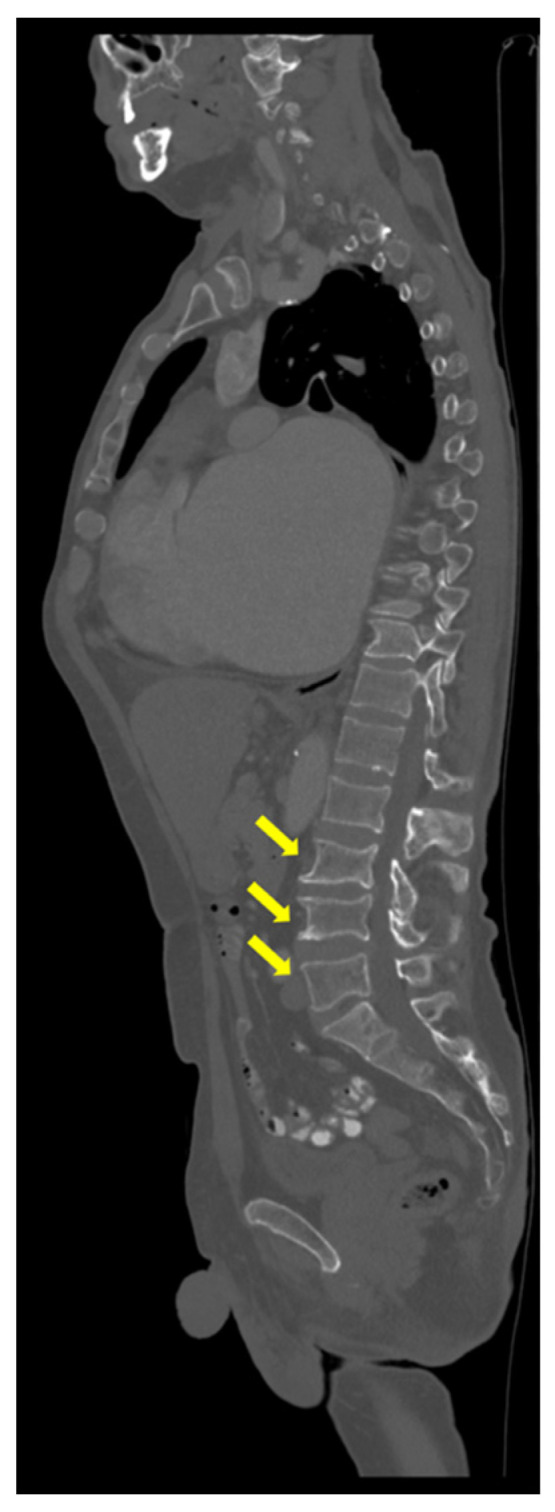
Native CT showing L3, L4, and L5 vertebral fractures (yellow arrow).
